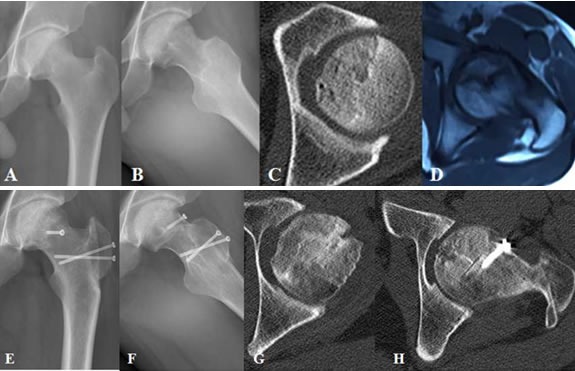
Thirty two-year male patient with alcohol-induced femoral head necrosis of left hip A. postoperative view (PA view) of left hip pre-operation; B. Frog position of left hip pre-operation; C. Necrosis area and collapse of femoral head (FH) shown in CT scan of left hip pre-operation; D. Crescent sign shown in MRI of left hip pre-operation; E. P-A view of left hip 6-months post-operation; F. Frog position of left hip 6-months post-operation; G. Bony healing of bone graft and no collapse of FH shown in CT scan 6-months post-operation; H. Bony healing of osteotomy in greater trochanter shown in MRI 6-months post-operation.
